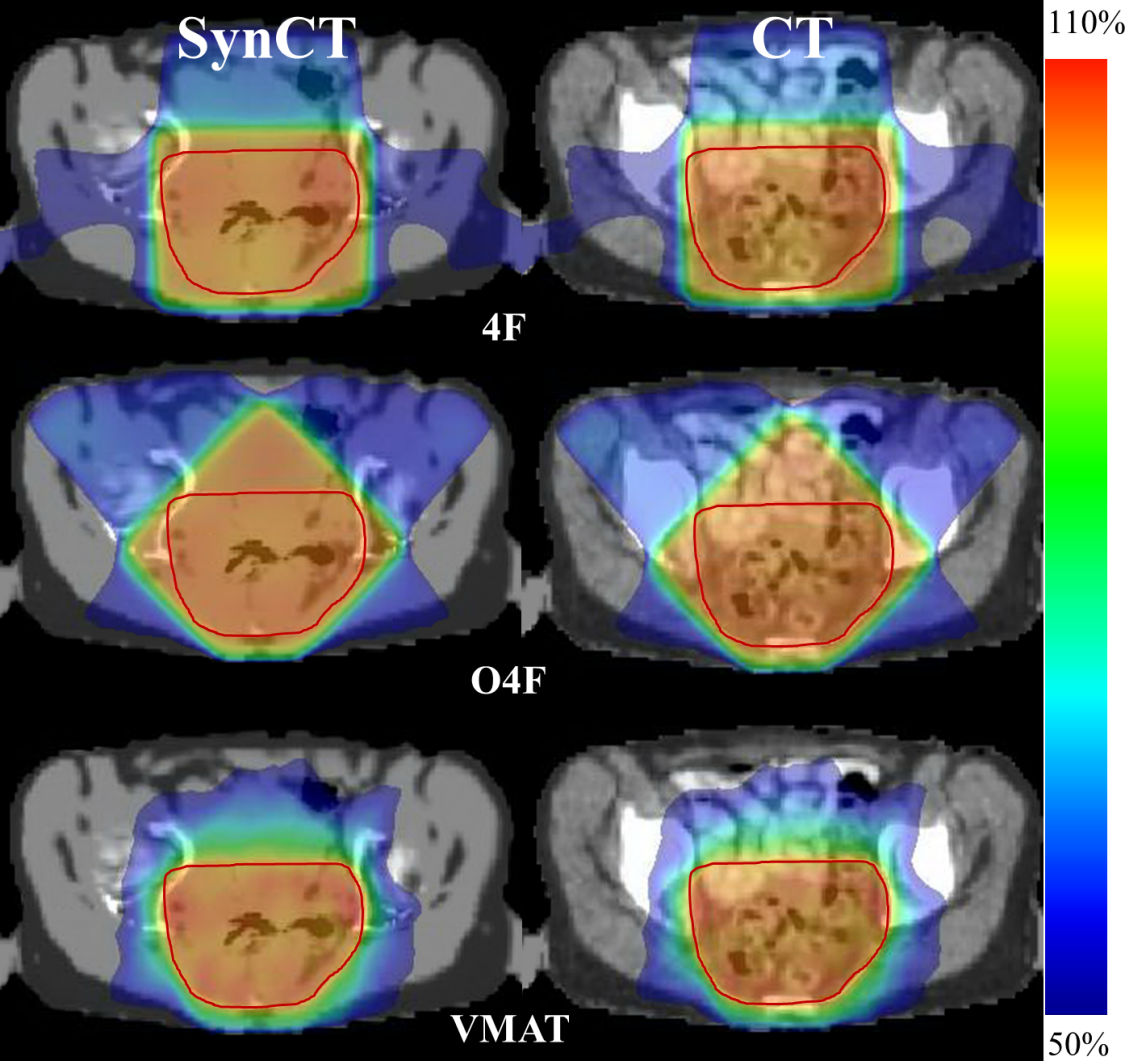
Doses of 4F (top), O4F (middle) and VMAT (bottom) plan overlapped on synCT (left column) and CT (right column). The doses are color mapped between 50% and 110% of prescription dose. PTV is contoured as red curve in color wash.
